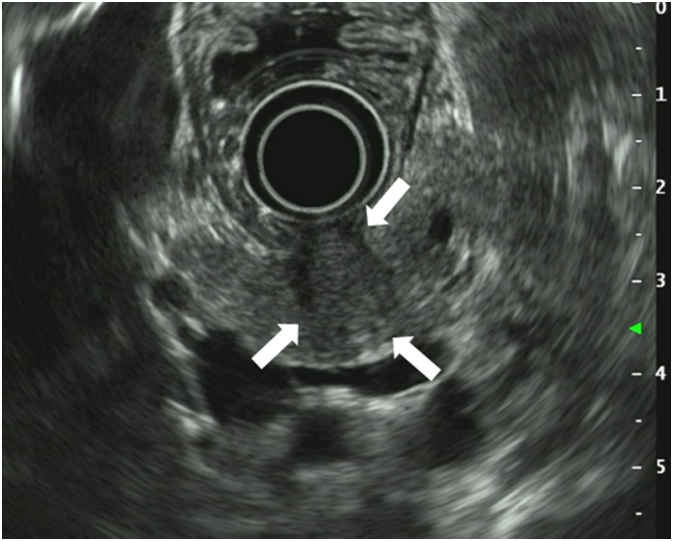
EUS scan findings. The tumor is visualized as a hypoechoic mass (white arrow) that measures 19 mm in diameter. EUS: endoscopic ultrasonography.
